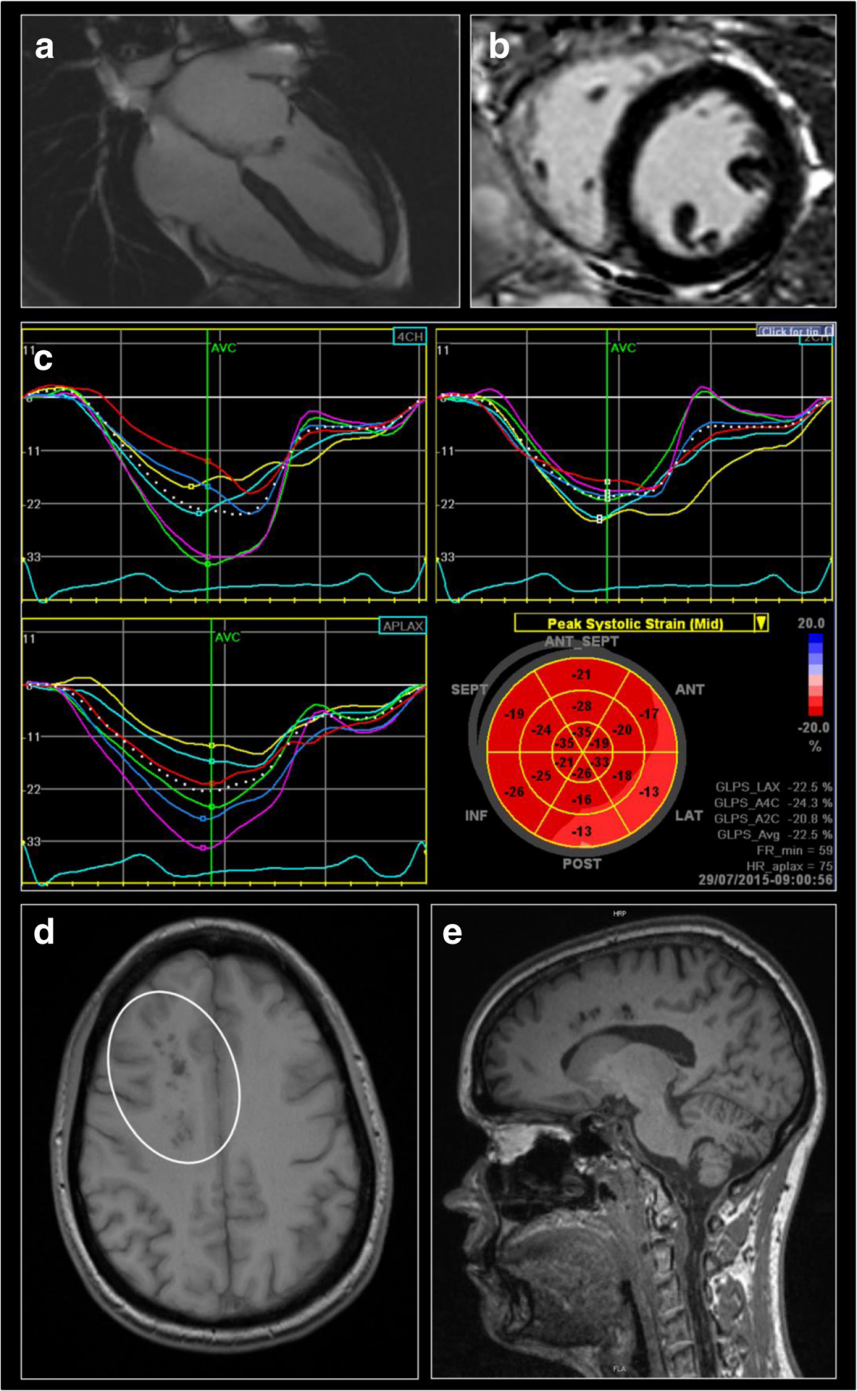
Index patient’s imaging features of cardiac a-c and brain d-e involvement at FAZIT baseline visit. Please note that morphologic and late gadolinium enhancement cardiac MRI a-b reveals mild hypertrophy and marginal fibrotic scar tissue with only minimal intramural late gadolinium enhancement detectable. In two-dimensional speckle tracking c peak systolic strain is mildly reduced, visualizing in loco typico early stage Fabry cardiomyopathy in the posterior-lateral and antero-lateral walls (bull’s eye method). Brain MRI d-e shows residual lesions (encircled) due to previously suffered cryptogenic stroke
